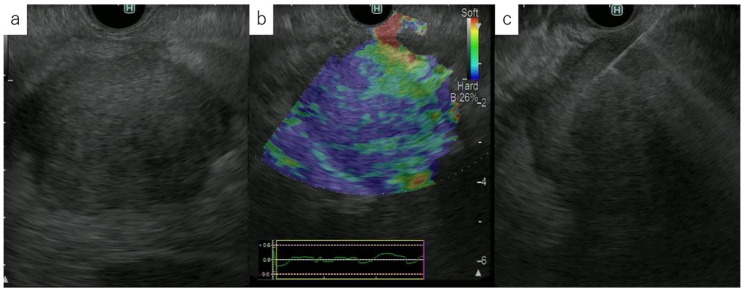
Targeting EUS-FNA with EUS-elastography. (a) Fundamental B mode endoscopic ultrasonogram showing a low echoic mass. (b) EUS-elastography image showing no distortion on low echo area and surrounding tissue. (c) EUS-FNA with EUS-elastography targeting the harder area.
